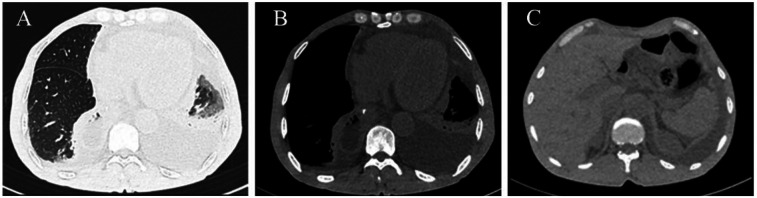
Chest CT findings. (A,B) Left medium pleural effusion. (C) Significant dilation of the colon.
